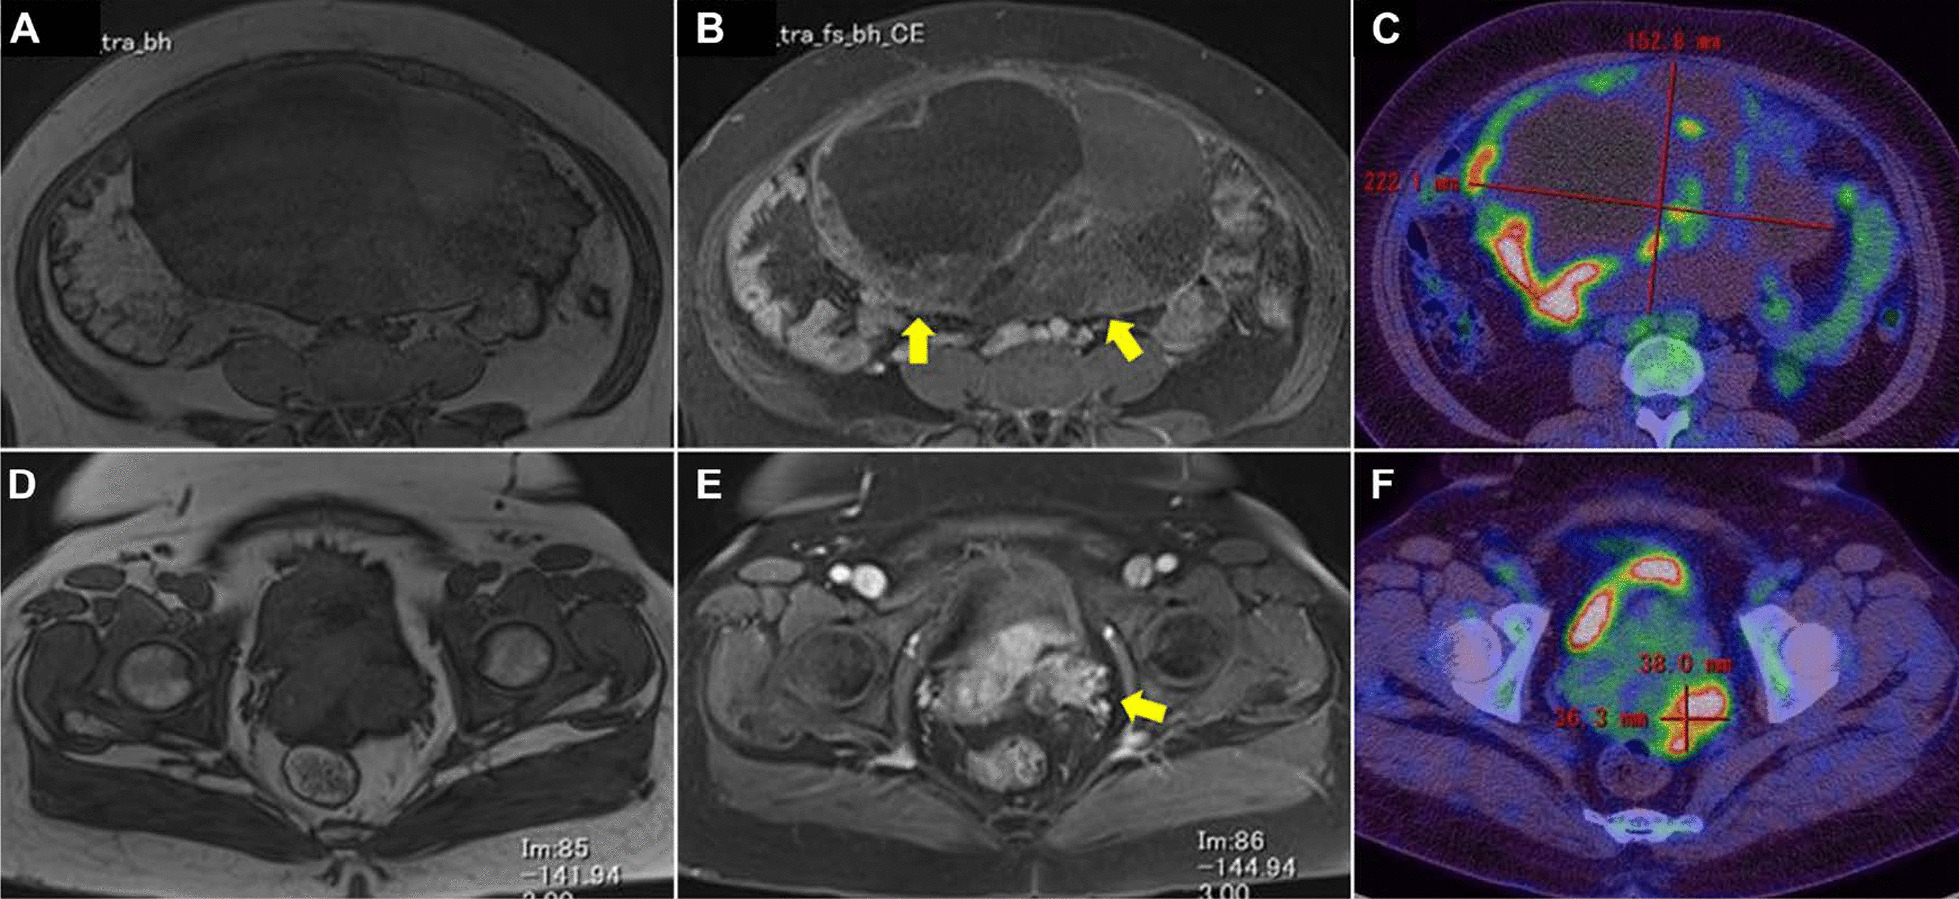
Images of MRI and PET-CT in the patient with synchronous bilateral ovarian tumor. Images of T1-weighted and T1-weighted gadolinium contrast enhancement in the right (A, B) and left (D, E) ovarian tumors. Images of PET-CT of the right (C) and left (F) ovarian tumors. Yellow arrows indicate contrast enhancement by gadolinium in the right and left ovarian tumor. *MRI* magnetic resonance imaging, *PET-CT* Positron-emission tomography and computed tomography
